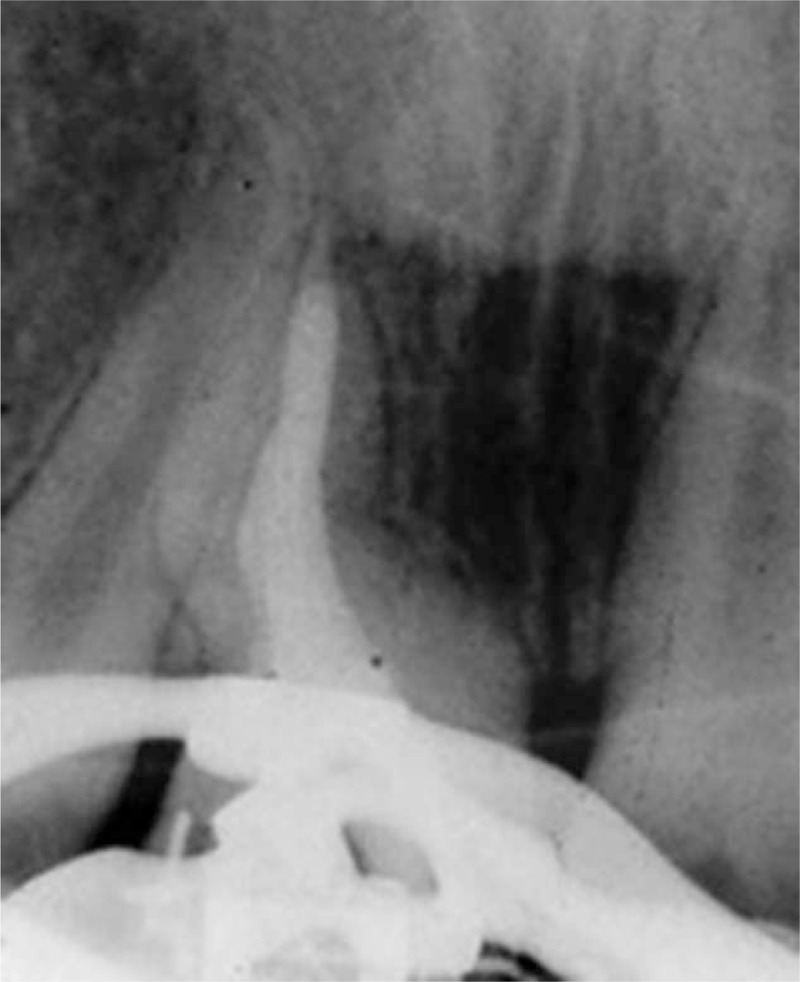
Final radiograph, control of the lateral condensation. It showed that the entire root canal space was filled with sealer and core material.
